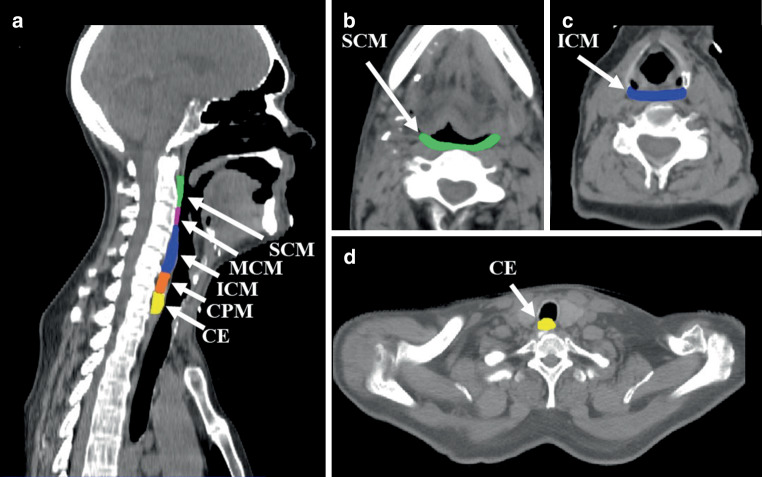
Defined muscular areas of the swallowing apparatus on a sagittal and b–d axial unenhanced CT slices. a gives an overview of the substructures of the swallowing apparatus, while b–d show selected swallowing structures. *SCM* Superior pharyngeal constrictor muscle, *MCM* Middle pharyngeal constrictor muscle, *ICM* Inferior pharyngeal constrictor muscle, *CPM* Cricopharyngeal muscle, *CE* Cervical esophagus
